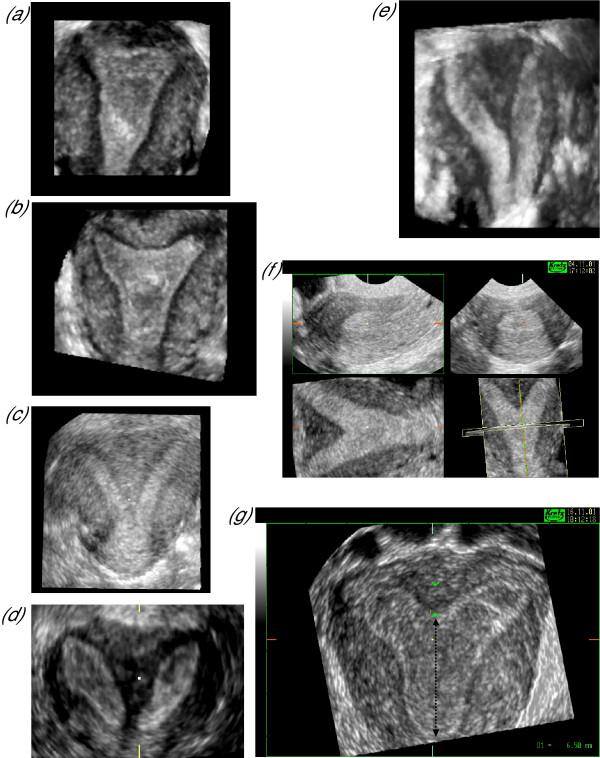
Uterine anomalies*. Three-dimensional sonography has become the 'gold standard' investigation for the diagnosis and exclusion of congenital uterine anomalies. Its extremely high sensitivity and specificity relate to its ability to demonstrate the plane coronal perpendicular to the transducer face and in doing so allow visualisation of the fundal contour and comparison of the myometrium with the endometrium throughout the uterine length. Figure 7a shows normal cornna with straight contours at the upper aspect of the cavity in contrast to the characteristic concave contour seen in arcuate uteri (Fig. 7b) and the deeper contour of various length seen in sub-septate uteri (Figs. 7c, 7d & 7e). Any indentation of the fundal contour may also be appreciated in the coronal plane as seen in the multiplanar display in Figure 7f. This uterus had been considered normal with conventional ultrasound, which only provides the longitudinal and transverse views seen in the upper two images, but laparoscopy had demonstrated a bulky uterus with a possible fundal defect and a follow-up three-dimensional ultrasound confirmed the presence of a significant septum. The size of the septal defect can be measured as shown in Figure 7g but may be less important than the remaining length of the cavity shown as a bold dashed line.
